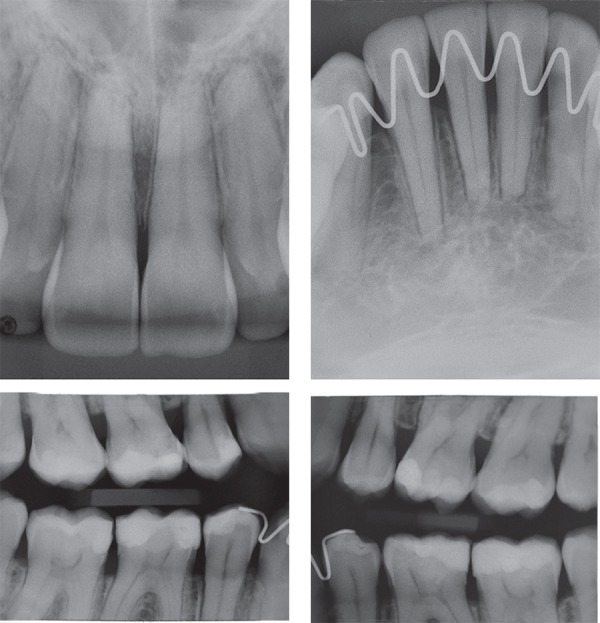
Final incisors periapical and interproximal radiographs.
